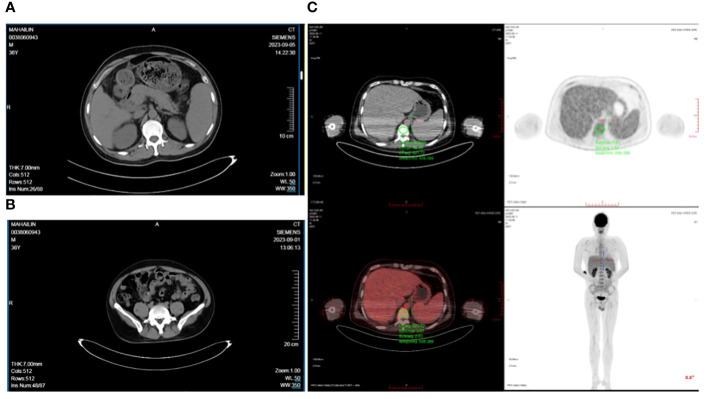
(A) The gallbladder wall was edematous, thickened, and rough with a slightly blurred surrounding fat space suggesting acute cholecystitis, (B) The appendix was slightly thickened, about 1.2 cm at its thickest, with a blurred surrounding fat space and multiple striated shadows, (C) PET-CT scans did not reveal any definitive signs of malignancy.
